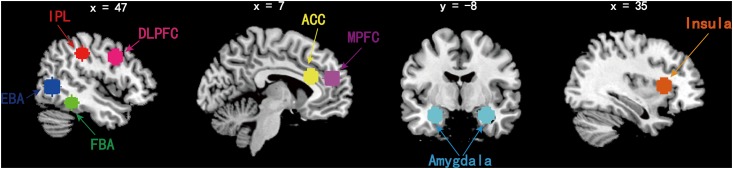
Sphere masks encompassing the IPL, EBA, FBA, ACC, MPFC, DLPFC, amygdala and insula used in ROI analyses of *f*MRI data. Red, inferior partial lobe (IPL); blue, extrastriate body area (EBA); green, fusiform body area (FBA); pink, dorsal lateral prefrontal cortex (DLPFC); yellow, anterior cingulate cortex (ACC); violet, median prefrontal cortex (MPFC); cyan, amygdala; orange, insula.
